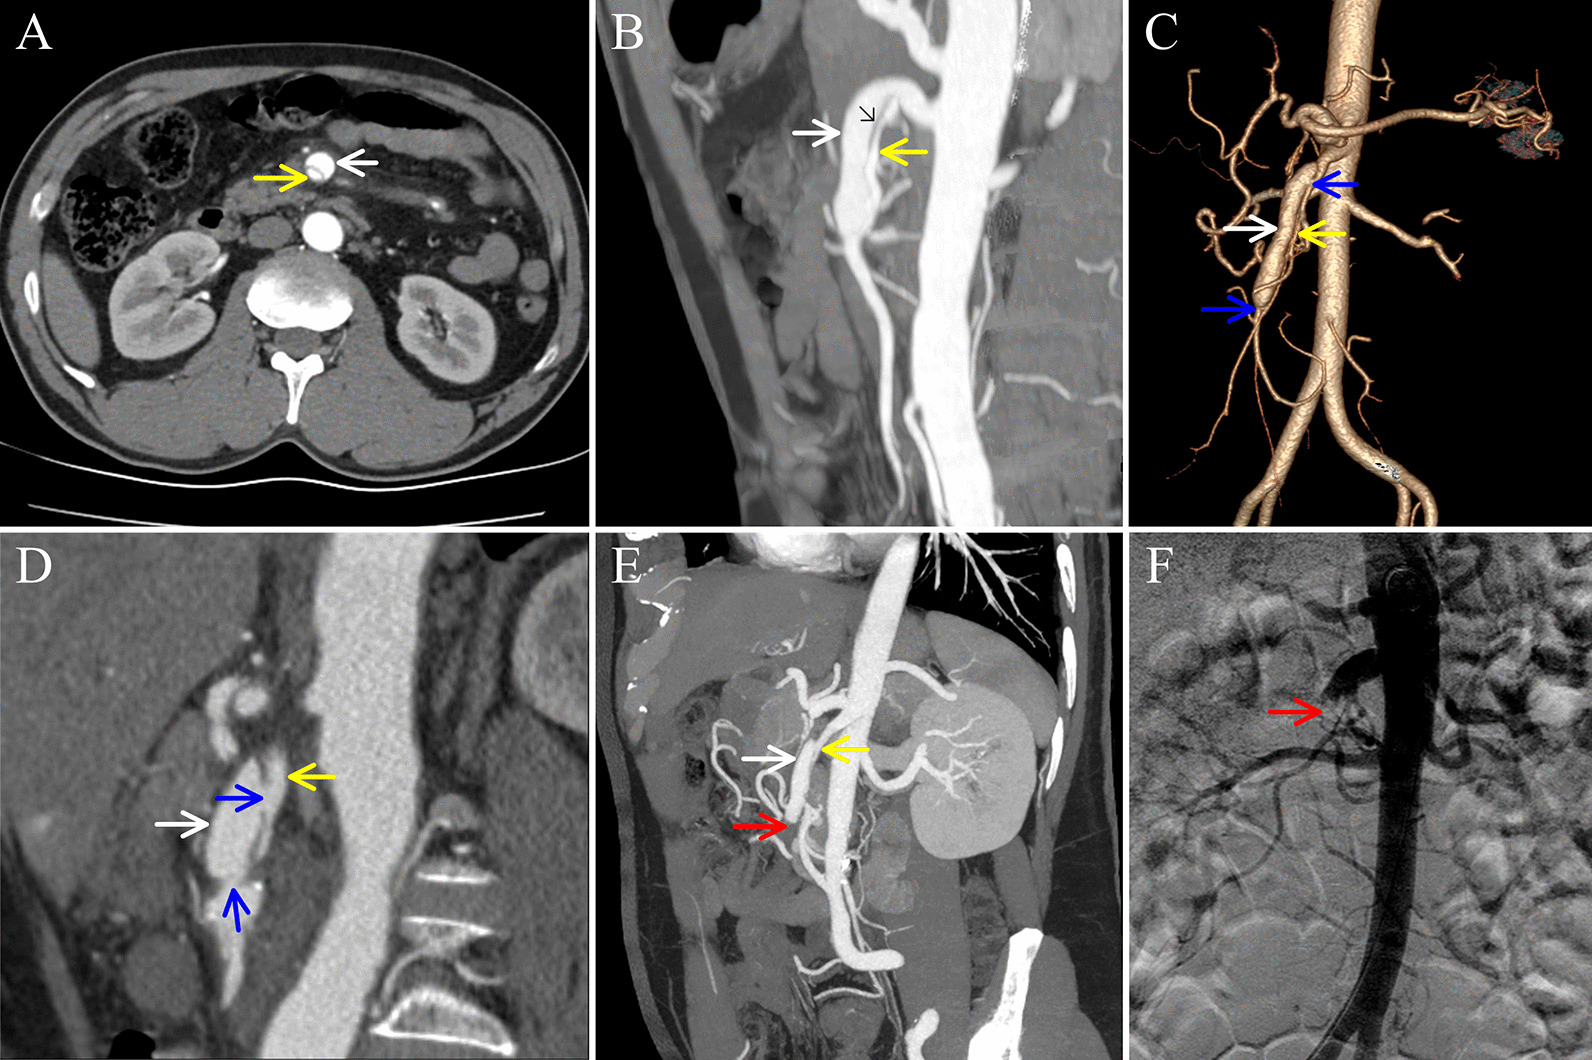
Features of Yun types I and III Dissection. (A) Axial CTA showing the characteristic finding of the double-lumen sign of the SMA below the LRV plane. (B) Sagittal CTA showing that the true and false lumens were separated by an intimal flap (black arrow). (C) CTA volume rendering showing the true and false lumens. (D) Longitudinal CTA showing the entry and re-entry sites. (E) Sagittal CTA showing complete occlusion of the SMA. (F) DSA showing complete occlusion of the SMA. The yellow arrow represents the true lumen; the white arrow represents the false lumen; the blue arrow represents the entry site or re-entry site; and the red arrow shows complete occlusion of the SMA. *CTA* computed tomography angiography, *SMA* superior mesenteric artery, *LRV* left renal vein, *DSA* digital subtraction angiography
